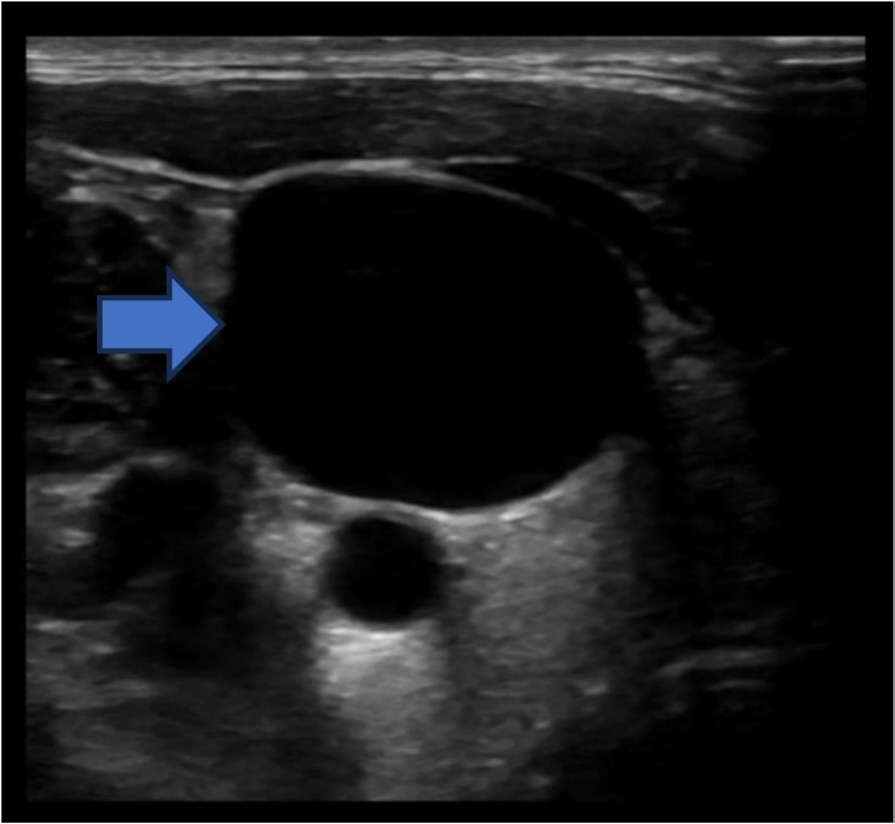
Point of ultrasound showing a very distended right internal jugular vein (blue arrow)
